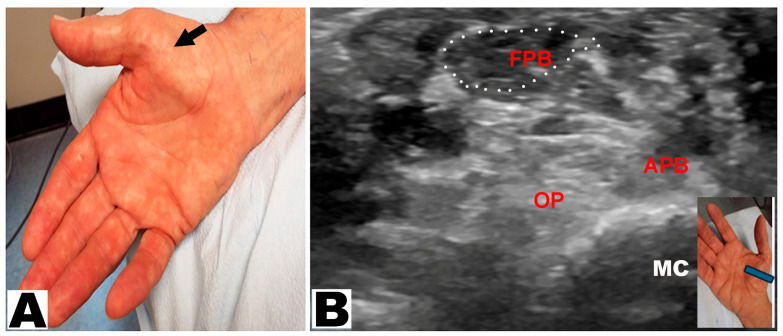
(A) Partial thenar atrophy with hypertrophy of the flexor pollicis brevis (FPB) muscle (oblique arrow). (B) Ultrasound study confirms partial thenar atrophy with a hypertrophic FPB muscle (encircled) and severe atrophy of the APB and OP muscles. The blue insert denotes the position of the ultrasound probe. APB: abductor pollicis brevis muscle. OP: opponens pollicis muscle. MC: metacarpal.
